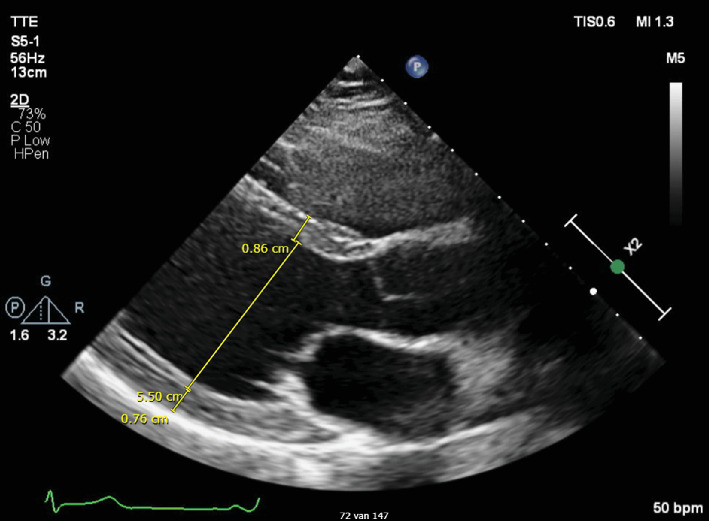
Echocardiography showing a mild dilated, nonhypertrophic ventricle.
